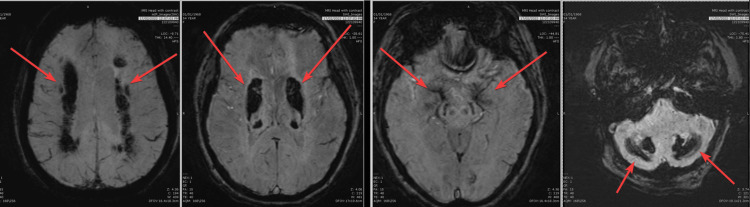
MRI susceptibility weighted imaging sequence demonstrates severe and intense calcification along the bilateral cerebellum, brain stem substantia nigra, thalamus, basal ganglia periventricular white matter, centrum semiovale, and occipital cortical gray matter.
